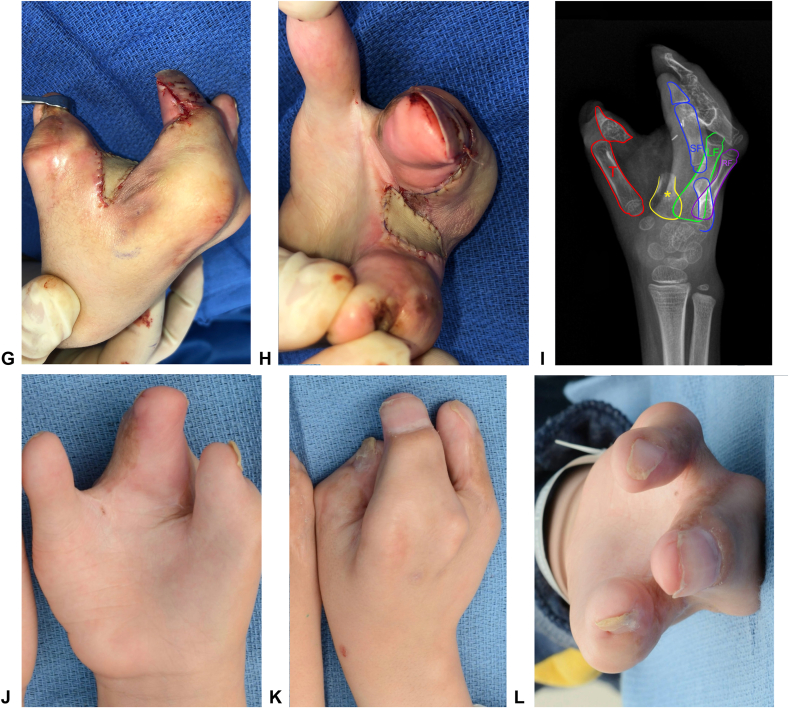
Example of a complex syndactyly case. The patient has Apert syndrome with Upton type three hands. They initially underwent first web space release with a dorsal trapezoidal flap and FTSG. However, the patient still had a tight first web space and limited active motion in the central three digits. Therefore, an index ray amputation was later performed to create a functional, deep first web space. A Preoperative clinical photo. B Preoperative x-ray. C Photo of the hand after the first stage release; note that the web space is still shallow. D, E Preoperative photos with surgical markings for the second stage surgery (index ray amputation). F X-ray of the hand after the first stage release. G, H Results after closure. I Postoperative x-rays. J–L Results at 4-month follow-up.
